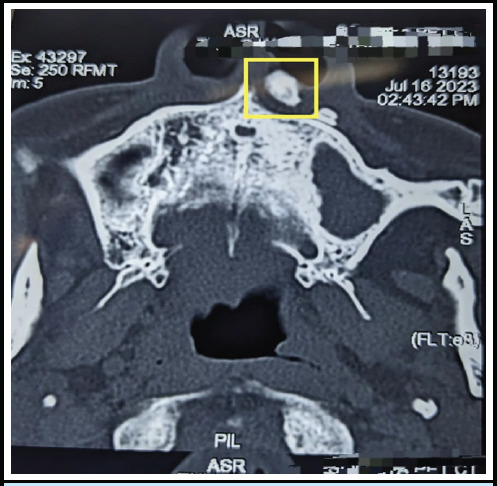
CT scan showing hyperdense focus in the left premaxillary soft tissue plane (inside yellow highlighted box).
